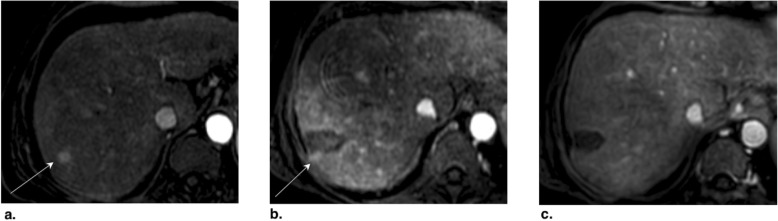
Axial T1-weighted fat-saturated MR in late arterial phase obtained (a) pretreatment: image shows nonrim arterial phase hyperenhancement (APHE) of a small HCC (arrow). b 1 month post radiofrequency ablation (RFA): image shows irregular rim enhancement and more nodular APHE at posterolateral margin (arrow). The treated observation is categorized LR-TR equivocal. c 4 months post RFA: image shows no suspicious enhancement; the treated observation is now categorized LR-TR Nonviable
